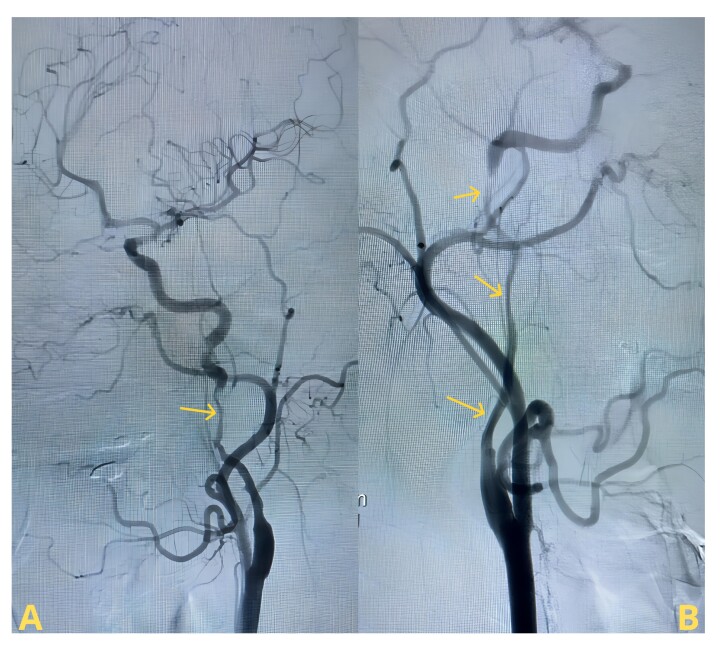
Cerebral arteriography image with internal carotid dissection. (A) Arrow indicates left carotid dissection; (B) Arrows indicate right carotid dissection. The left carotid bulb occlusion suggested in Figure 1A was not visible on arteriography, being only apparent on the previous angio-CT study.
